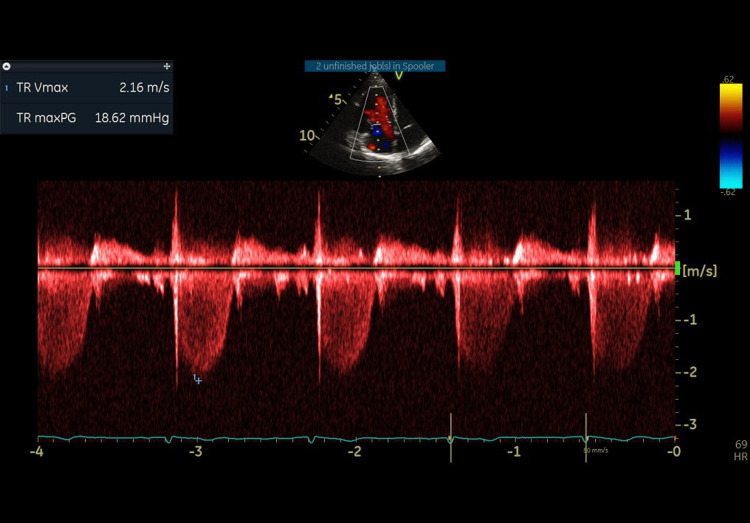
A continuous wave-Doppler across the tricuspid valve in severe tricuspid regurgitation
